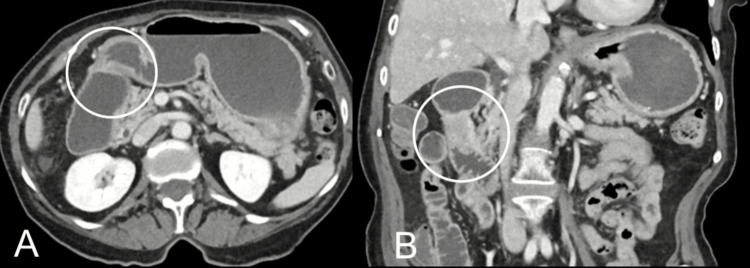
CT abdomen and pelvis with contrast (A) CT scan showing gastric outlet obstruction (transverse plane). (B) CT scan showing gastric outlet obstruction in the second part of the duodenum (coronal plane).
